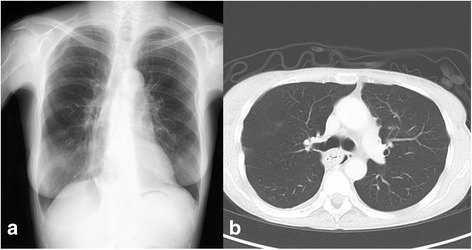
Chest radiography (a) and computed tomography (b) images before discharge. a Reexpanded right lung and gastric conduit on the mediastinal field are seen. b Reexpanded right lung, disappearance of pleural effusion, and gastric conduit on the right posterior mediastinum are seen
